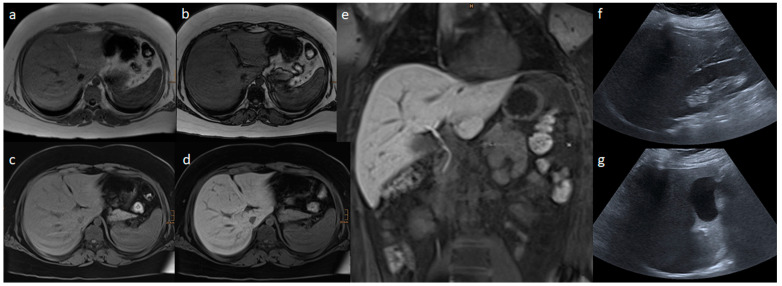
Liver MR and ultrasound images of a 13.7-year-old boy with a body mass index of 40.1 kg/m^2^: (a) In-phase; (b) opposed phase; (c) non-contrast T1 VIBE fat saturated; (d,e) transitional phase of Gd-EOB-DTPA-enhanced T1 VIBE fat saturated sequences; (f) AAL; and (g) MCL liver ultrasound images. In the ultrasound, a mild steatosis hepatis was estimated based on the echogenicity of the liver parenchyma. In the MRI, the liver volume was 1953 mL, and the hepatic fat fraction was 15.9% based on moderate steatosis hepatis, and 1.3 was the relative enhancement.
